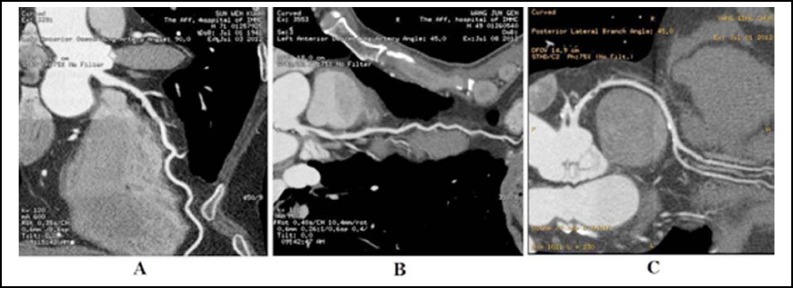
64-slice spiral CT images of luminal stenosis induced by plaques. A: mild stenosis of middle LAD; B: moderate stenosis of middle LAD; C: severe stenosis of proximal and middle RCA
